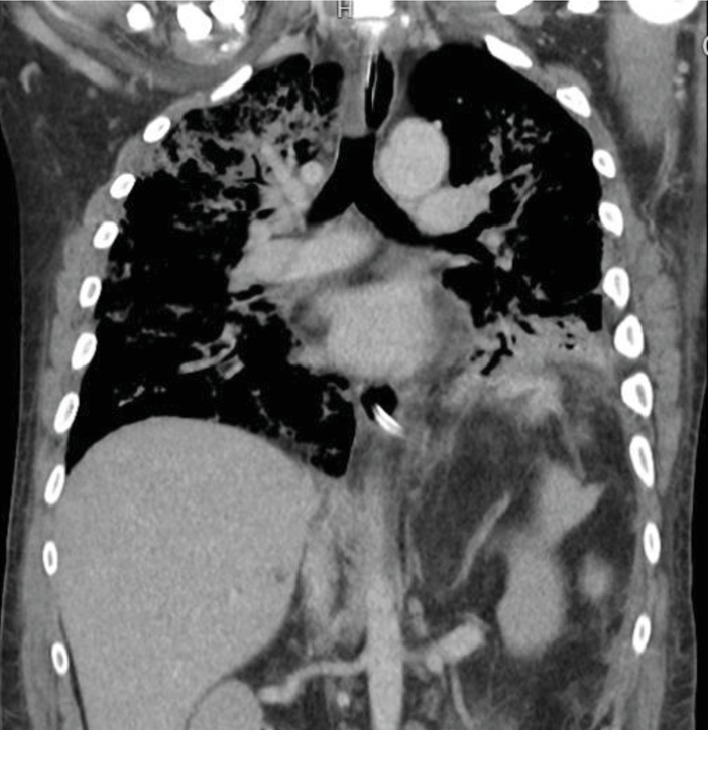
CT scan of left lower lobe lung injury secondary to the gastrobronchial fistula and oral contrast within the lung.
